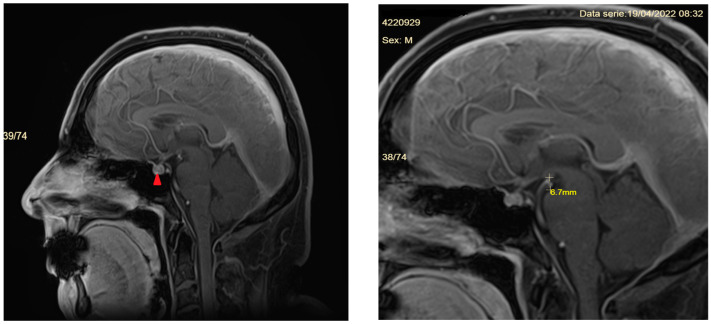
Sagittal T1-weighted contrast-enhanced images of the patient’s brain. The picture on the left shows the pituitary enlargement and hyperemia (red arrow), while the one on the right highlights the reduced mammilopontine distance (6.7 mm). Although normally, this distance is measured in sagittal non-enhanced T1 images we could retrieve only enhanced T1 sagittal sections in the imaging study.
